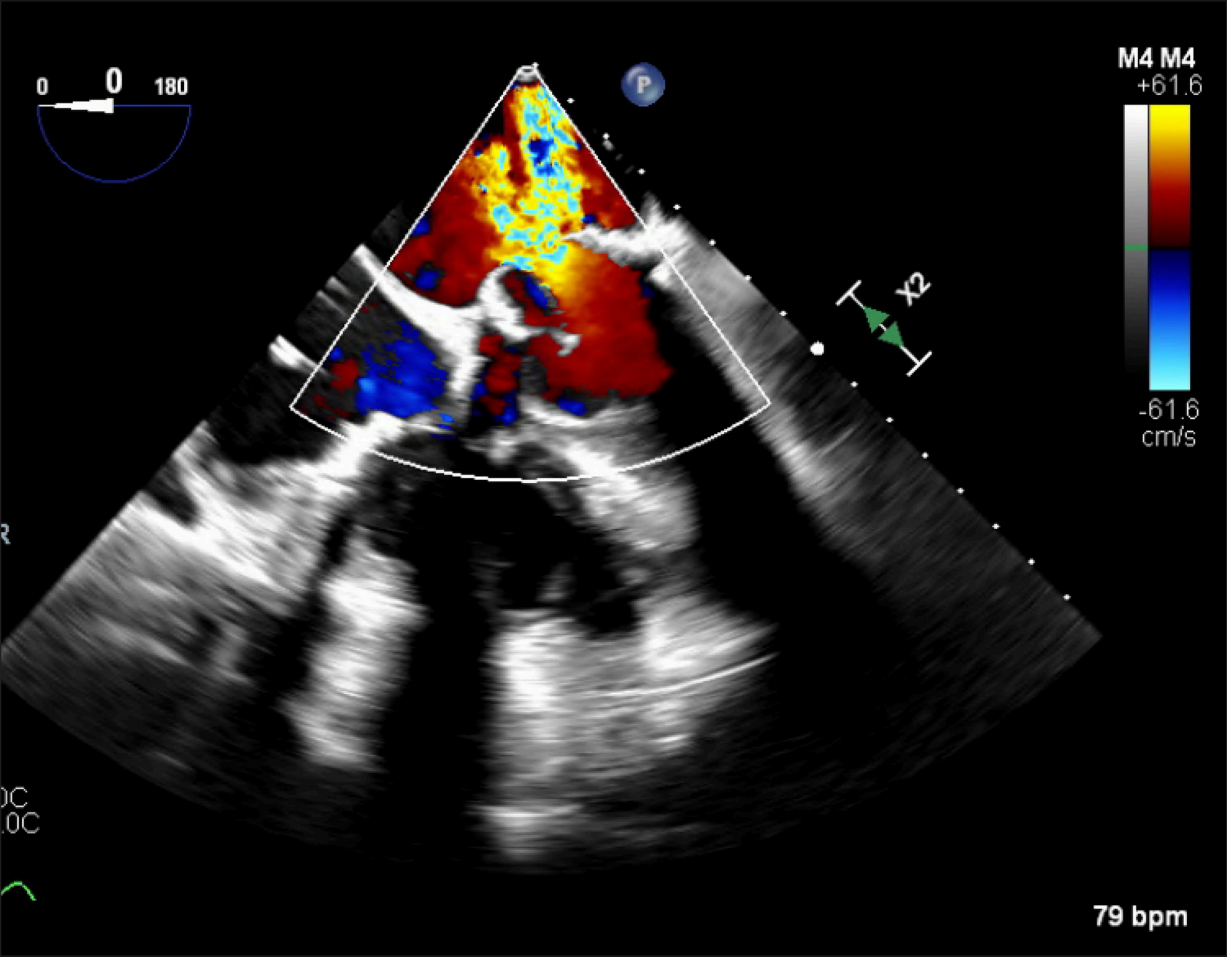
Transesophageal echocardiogram showing severe mitral regurgitation.
